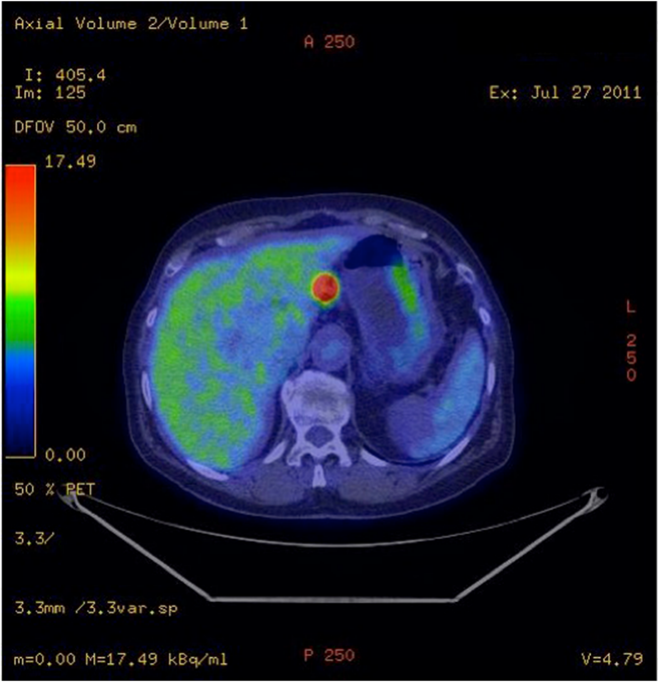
Positron emission tomography/computed tomography revealed a mass in the pancreatic head with metabolic activity.
